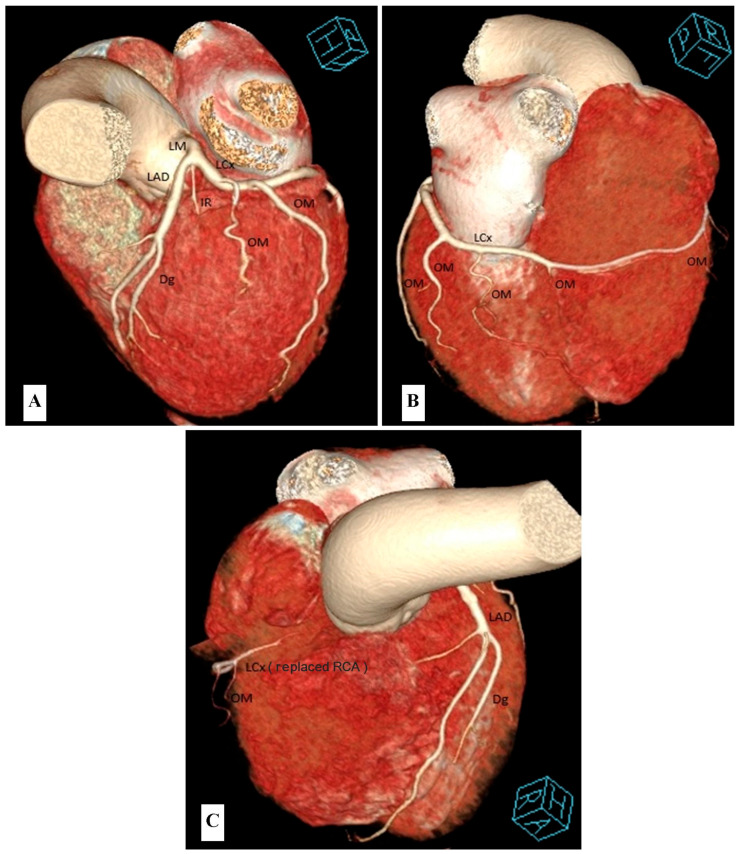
Cardiac computed tomography angiography. Angiographic phase. Volume Rendering Technique (VRT) reconstruction. Coronary circulation—single coronary artery. LM—left main coronary artery, LAD—left anterior descending artery, Dg—diagonal branch, IR—intermediate branch, LCx—left circumflex artery, OM—obtuse marginal branch, RCA—right coronary artery. (A) cranial-posterior-left oblique view. (B) caudal-posterior-right oblique view. (C) cranial-anterior-right oblique view.
